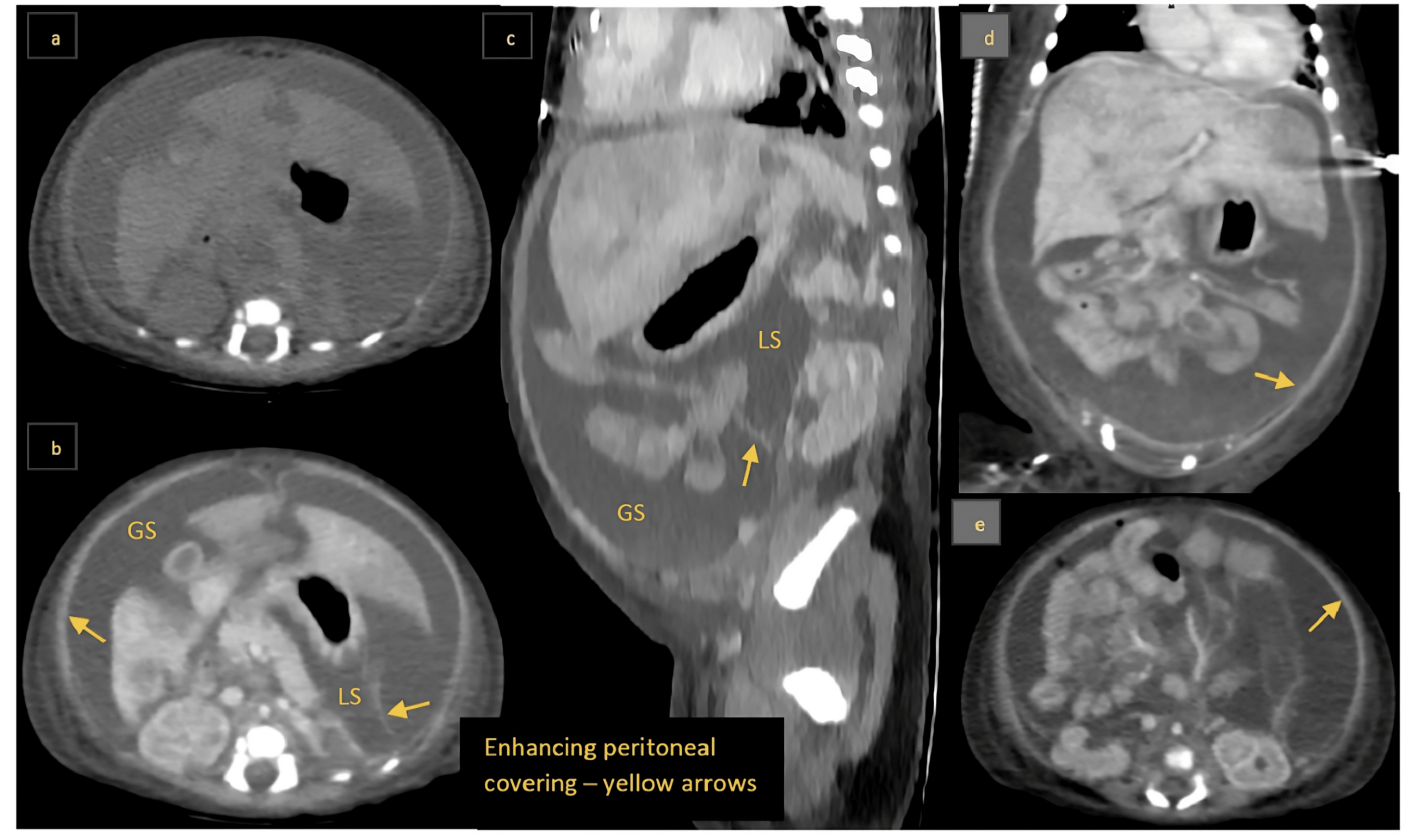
a: Axial non-contrast CT showing gross ascites. b: Axial contrast-enhanced CT showing peritoneal enhancement. c: Sagittal contrast-enhanced CT reconstruction showing loculated ascites in the greater and lesser sacs. d: Coronal contrast-enhanced CT showing clumped small bowel loops with peritoneal enhancement. e: Axial contrast-enhanced CT showing peritoneal encapsulation around the clumped small bowel. CT: computed tomography, GS: greater sac, LS: lesser sac
